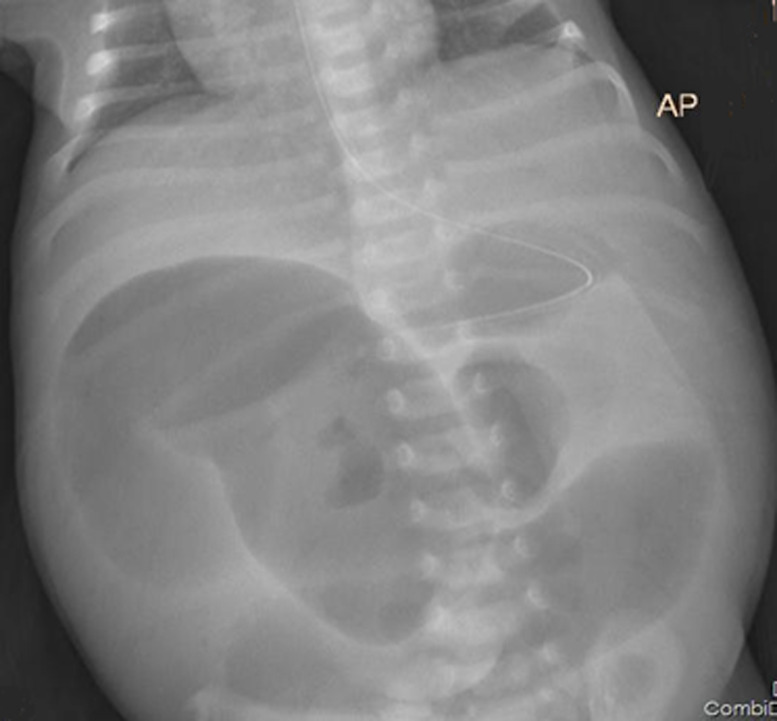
supine pre-operative X-ray which shows dilated small bowel
